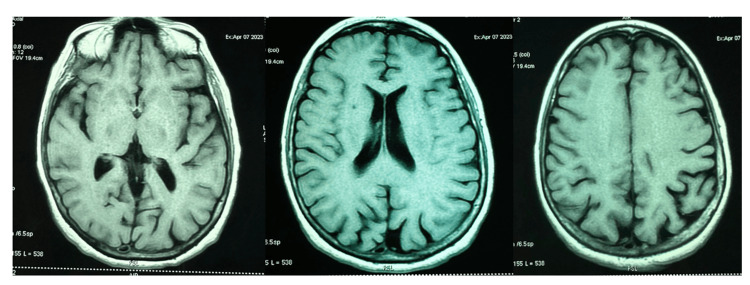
Magnetic resonance imaging (MRI) of the brain, axial T1-weighted image, showing no abnormalities
